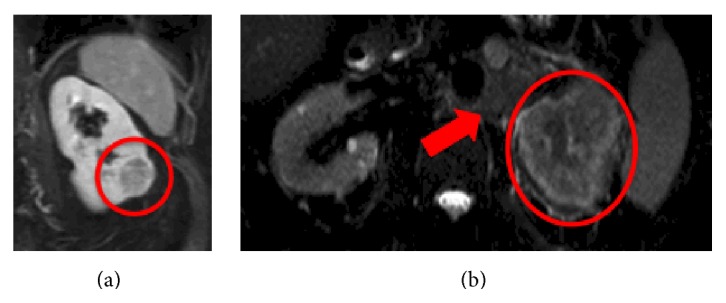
Conventional MRI provides anatomic but not physiologic information about kidney tumors. (a) 3 cm exophytic renal mass is imaged with conventional MRI that can only provide information about the size of a renal mass and its enhancement after administration of gadolinium-based contrast agent. Based on its size, there is a 30% likelihood that it is benign. Percutaneous core needle biopsy determined that it was a renal cell carcinoma. (b) 7 cm endophytic renal mass with para-aortic lymphadenopathy indicated by the red arrow.
